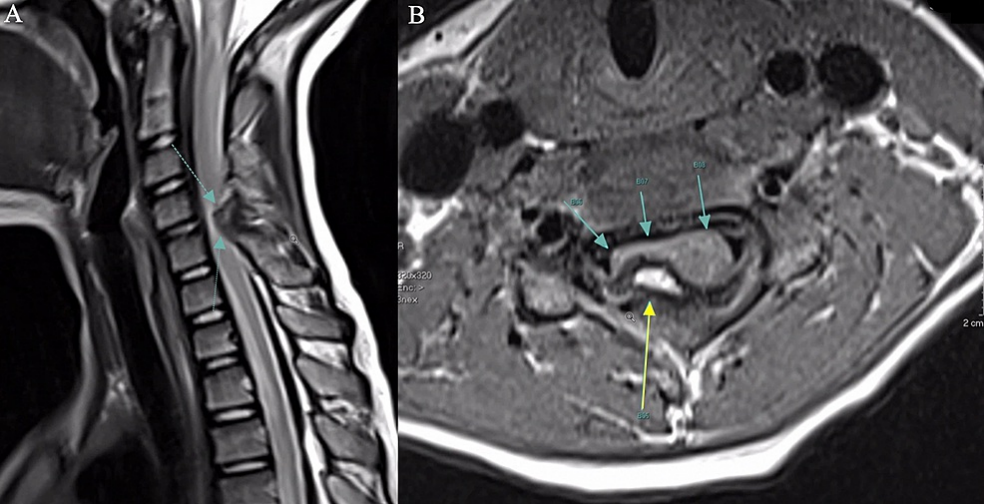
MRI cervical spine T2-weighted sagittal view (A) denoting bony expansile lesion with extension into the spinal canal (dashed arrow). MRI cervical spine T1-weighted axial view (B) showing bony lesion (solid blue arrows) and high-grade compression of the right aspect of the spinal cord (yellow arrow).
